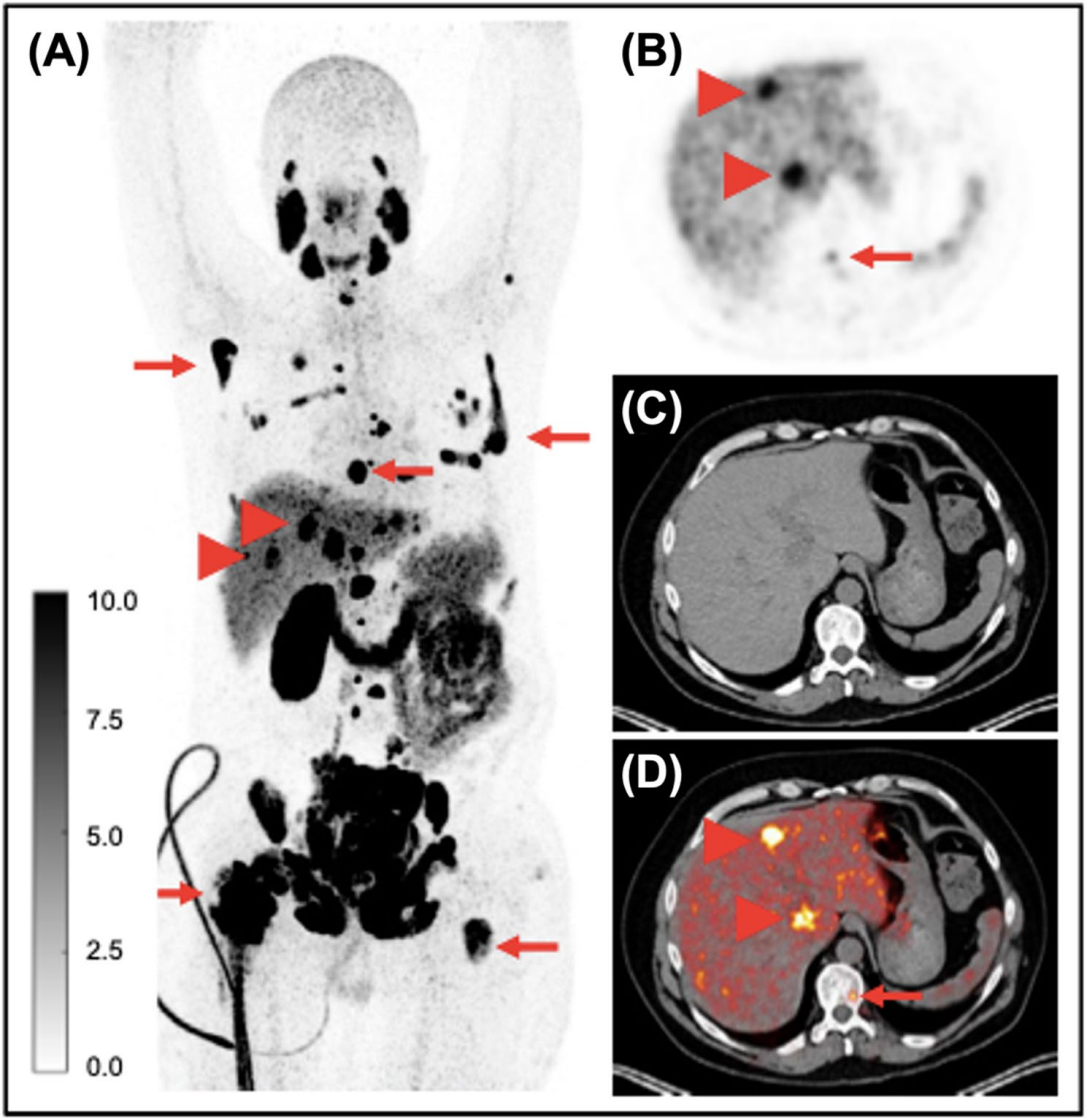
PET/CT images acquired 3 h after administration of [^61^Cu]Cu-NODAGA-PSMA-I&T in a 48-year-old patient with metastatic castration-resistant PCa, following disease progression after abiraterone and docetaxel treatment and prior to [^177^Lu]-PSMA radioligand therapy. Maximum-intensity projection (A), PET (B), CT (C), and fused PET/CT (D) images show multiple osseous metastases (arrows) and hepatic metastases (arrowheads). The patient had a history of left nephrectomy. Reproduced from Bernabeu et al. [106], licensed under CC BY 4.0 (https://creativecommons.org/licenses/by/4.0/)
